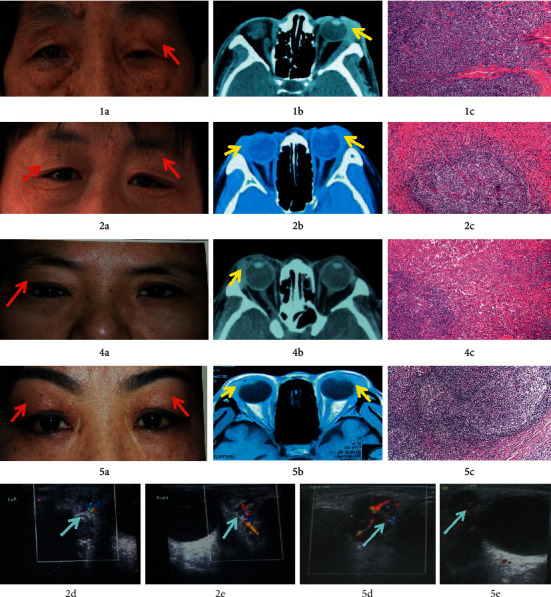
Case 1: 1(a) ∼ 1(c), case 2: 2(a) ∼ 2(e), case 4: 4(a) ∼ 4(c), and case 5: 5(a) ∼ 5(e). Preoperative appearance of the eyes (red arrows in 1(a), 2(a), 4(a), and 5(a)). CT/MRI showed swollen masses in the lacrimal gland area (yellow arrows in 1(b), 2(b), 4(b), and 5(b)). Pathological images (1(c), 2(c), 4(c), and 5(c)). Color ultrasonography showed masses in the lacrimal gland area with abundant blood flow (blue arrows in 2(d), 2(e), 5(d), and 5(e)).
